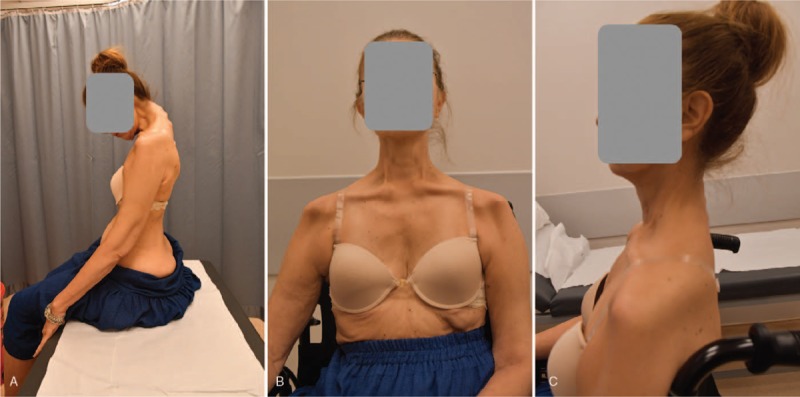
Postoperative X-rays (A and B) and computerized tomography scan (C) showed successful union.
